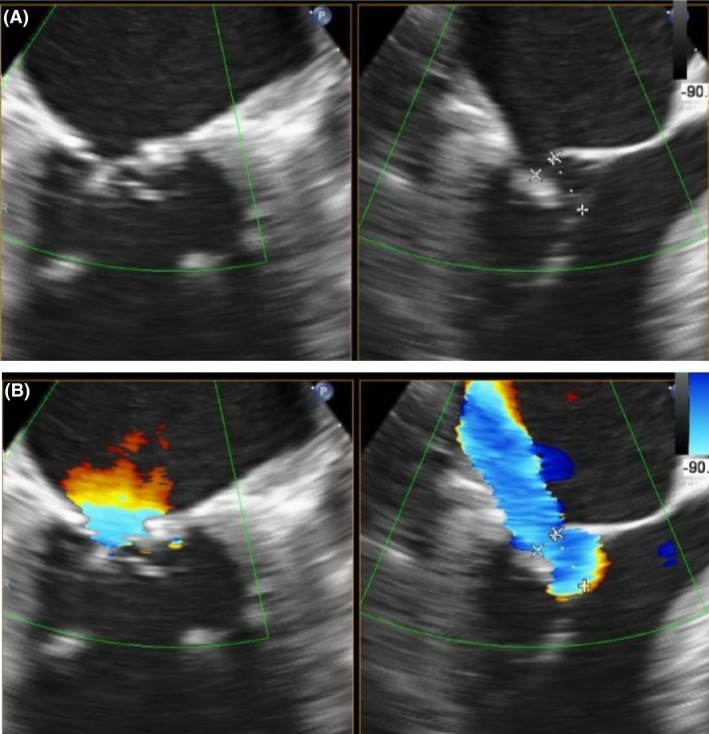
Transesophageal echocardiography showing the native mitral valve before intervention. Two‐chamber view (left) and left ventricular outflow tract view (right) without (A) and with (B) color Doppler encoding. The calcified and restrictive posterior leaflet leading to malcoaptation can be seen.
